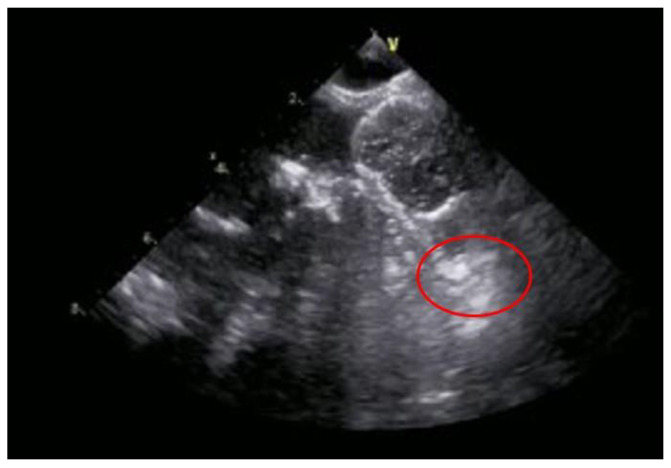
Transesophageal echocardiography image of LAAO with Watchman FLX in the presence of LAA thrombosis.
